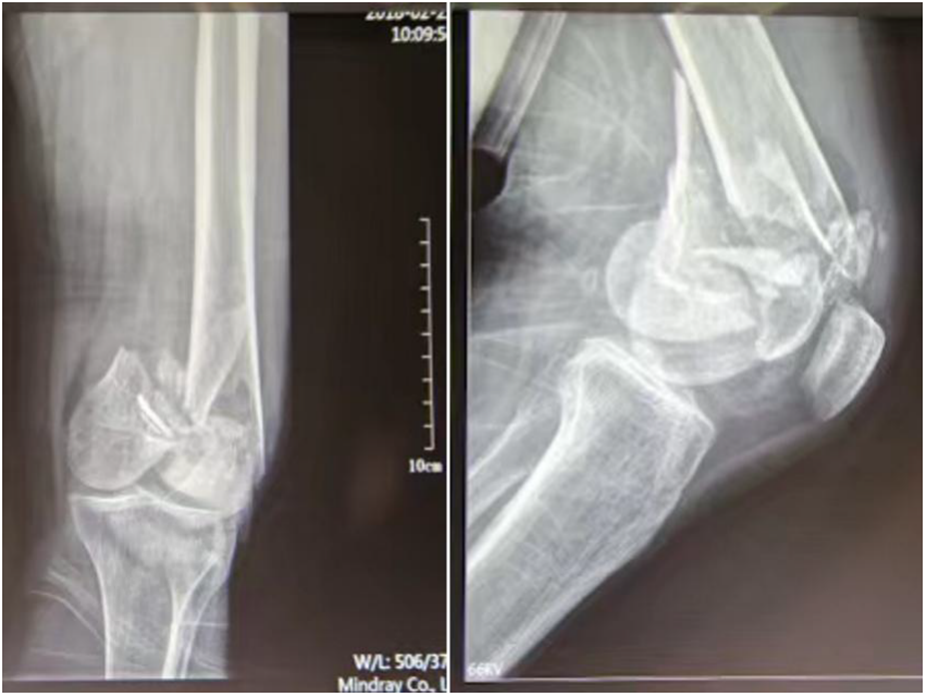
Preoperative x-ray of intercondylar supracondylar fracture of the left femur.
